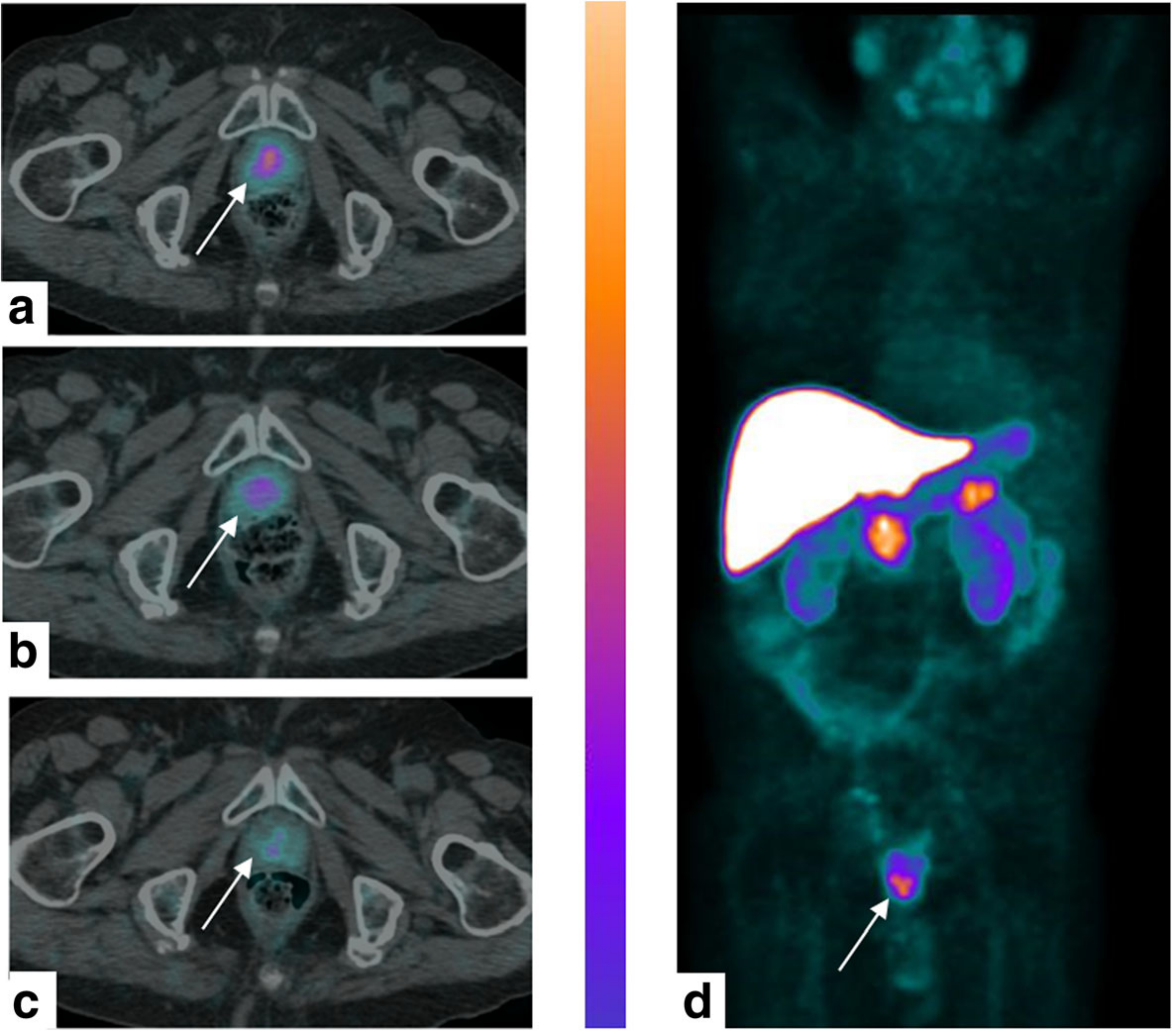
An 85-year-old man affected by Gleason 4+3 PCa treated with EBRT, with rising PSA level (6.0 ng/mL) and PSA doubling time of 15 months. ^64^CuCl_2_-PET/CT images (axial and MIP) revealed focal and pathologic tracer uptake (arrows) in correspondence to the prostate apex close to the midline 1 h after the injection (a). An important reduction of tracer uptake was observed 4 (b) and 24 h (c) after the injection. Maximum intensity projection (d) visualized the differences in terms of uptake between the organsand prostate relapse
